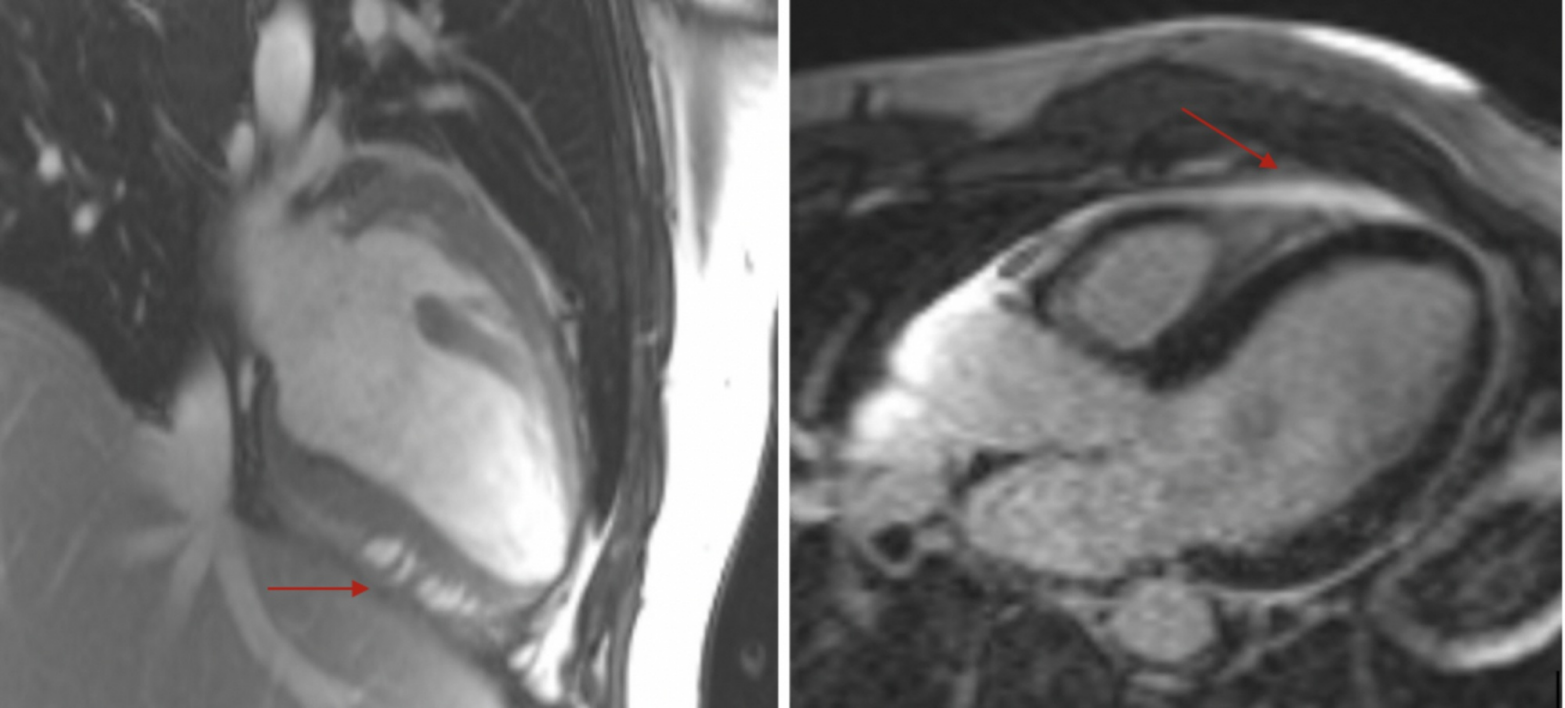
Cardiac MRI showing early (left arrow) and late (right arrow) gadolinium enhancement consistent with myocarditis MRI, magnetic resonance imaging
